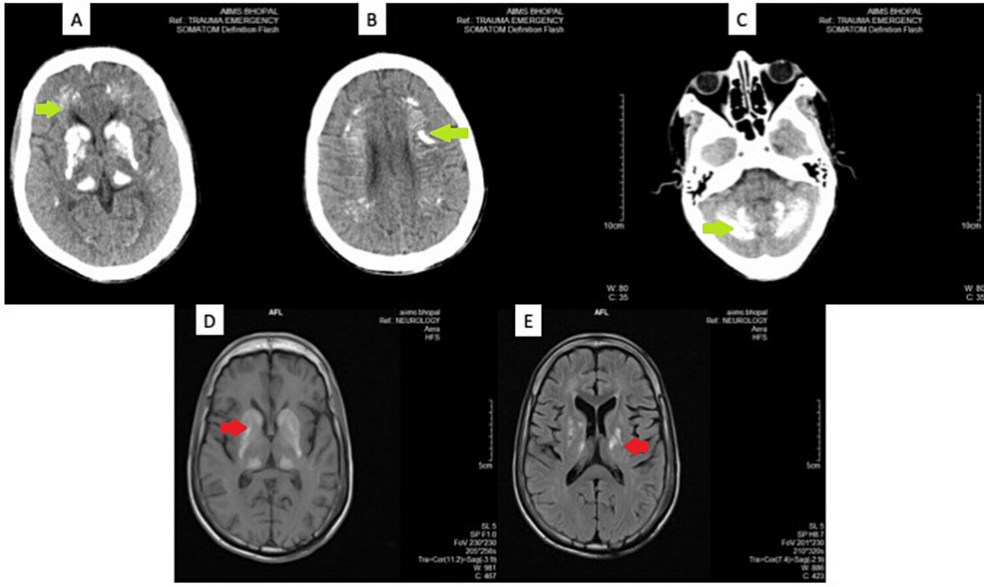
Non-contrast CT and MRI images showing symmetrical areas of bilateral intracranial calcification (A)-(C) are NCCT images showing symmetrical areas of calcification (green arrows) in bilateral basal ganglia and thalami, head of caudate nucleus, lentiform nucleus, and thalamus (A), periventricular white matter (B), and cerebellar hemispheres (C). (D) and (E) are corresponding MRI images showing symmetrical areas of altered signal intensity (red arrows) involving bilateral basal ganglia, thalami, periventricular and deep white matter, corona radiata, centrum semiovale (D). There also are multiple small confluent areas of gliosis in bilateral centrum semiovale, suggestive of old infarcts (E).
